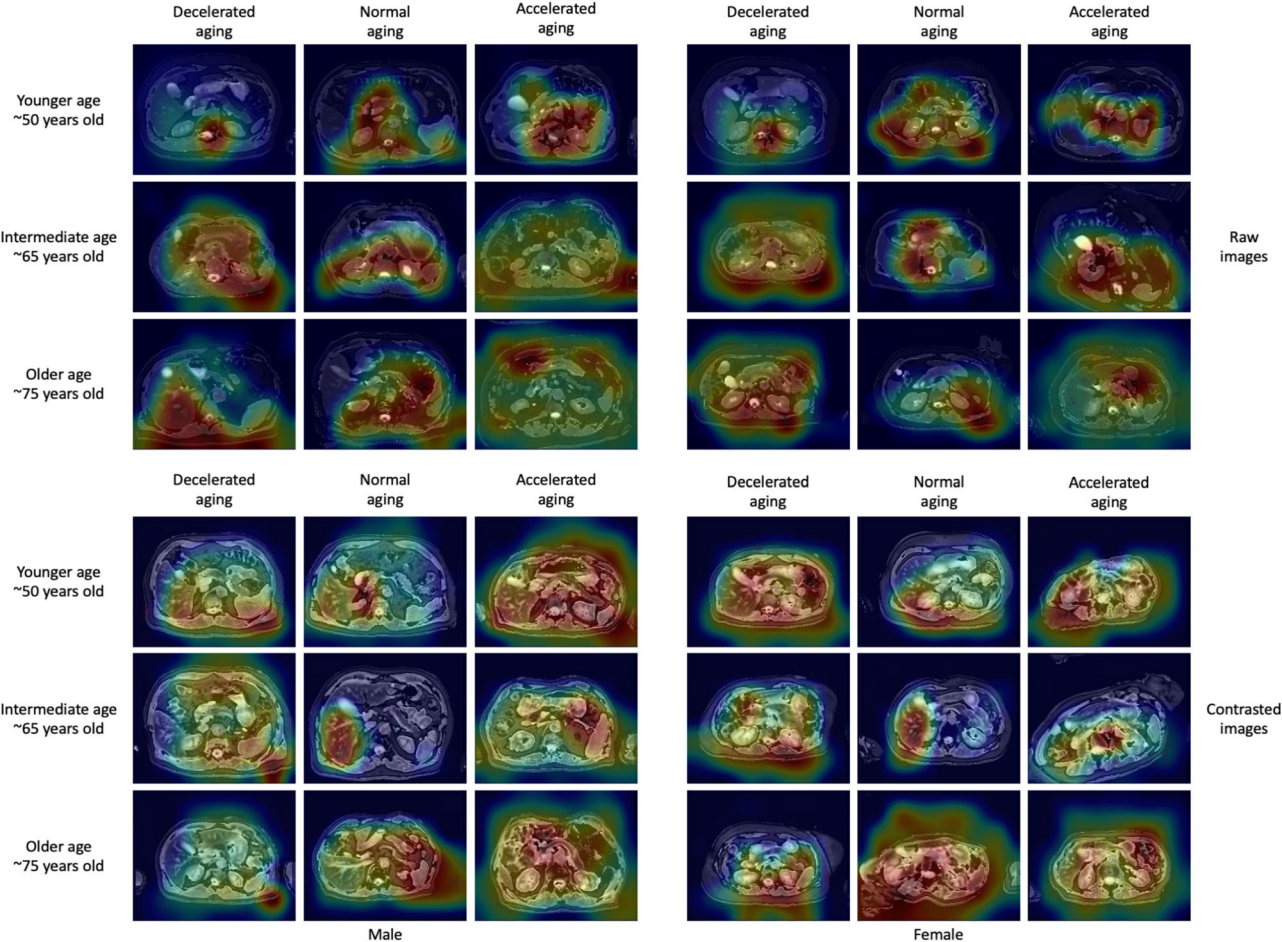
Sample attention maps for pancreas MRI-based models on raw and contrasted images. “Warm” filter colors (more red) highlight regions of high importance according to the Grad-RAM map. Actual chronological ages are obscured.
